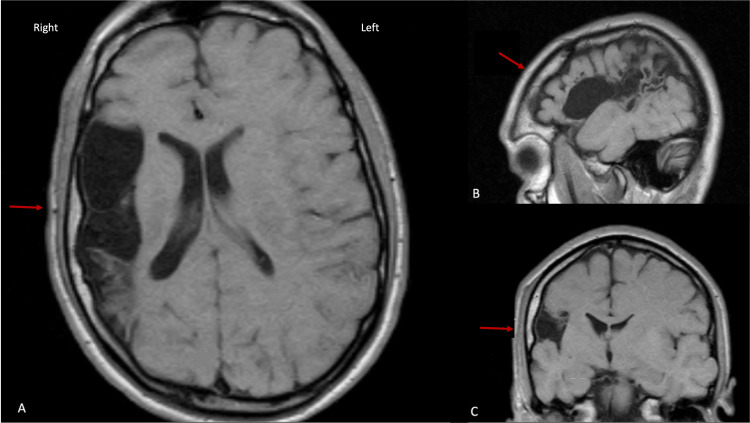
MRI brain (T1 weighted). Panel A: Axial. Smaller right hemisphere compared to the left secondary to intrauterine stroke on axial view. There is ipsilateral thickening of the skull (arrow) resulting in a Dyke-Davidoff-Masson syndrome. Panel B: Parasagittal view. Panel C: Coronal view.
